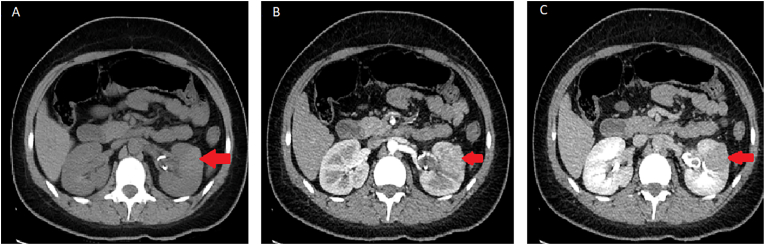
Enhanced CT scan of left kidney lesion in (A) non contrast phase, (B) arterial phase, and (C) nephrogenic phase. The red arrows point at kidney mass, which shows enhancement of the lesion with contrast. *The mass originates from the cortex measures 30 × 25 × 35 mm in the mid third of the left kidney. (For interpretation of the references to colour in this figure legend, the reader is referred to the Web version of this article.)
